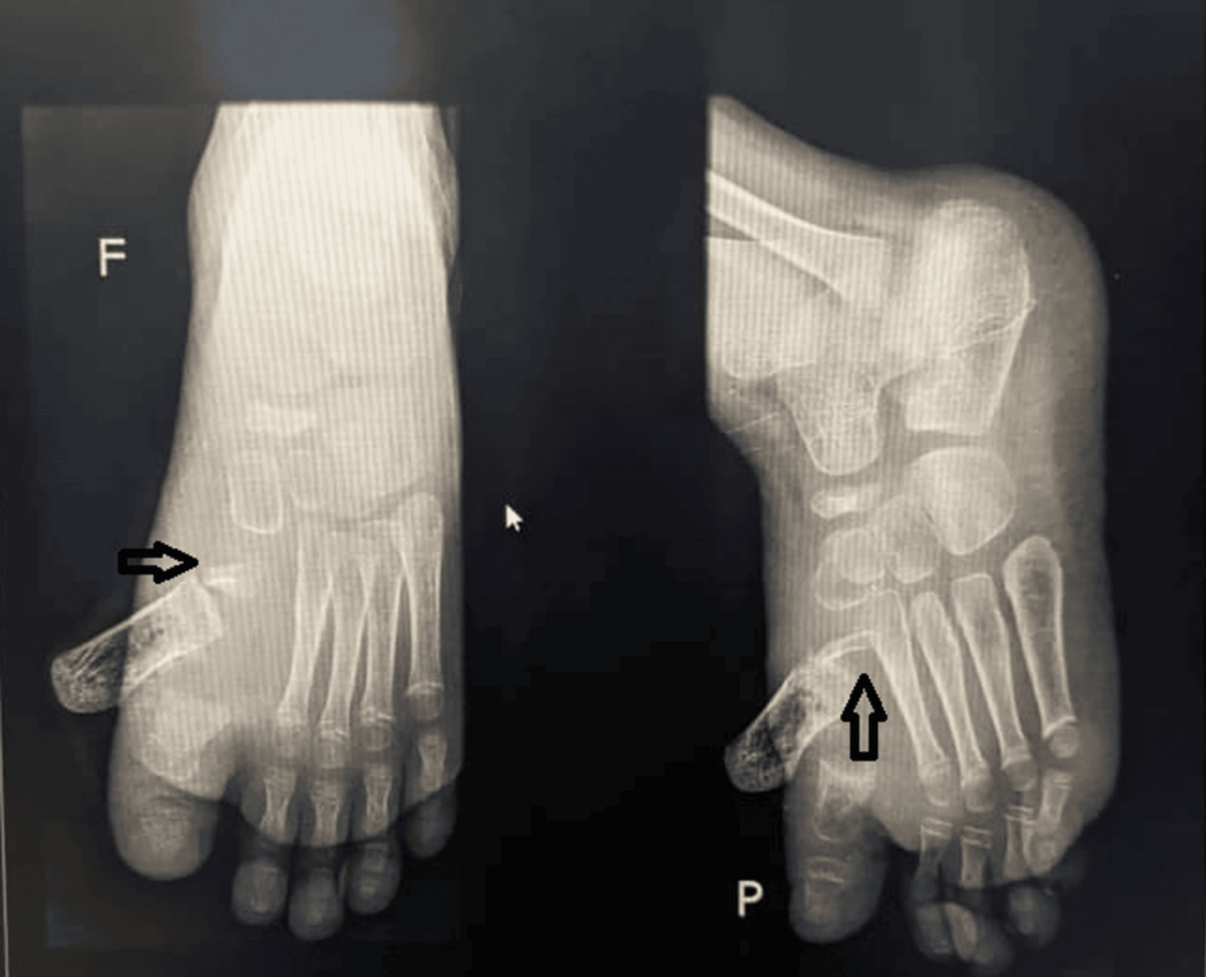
Left foot X-ray Salter-Harris type 1 fracture of the left first metatarsal (black arrow) (F: front view, P: side view)
